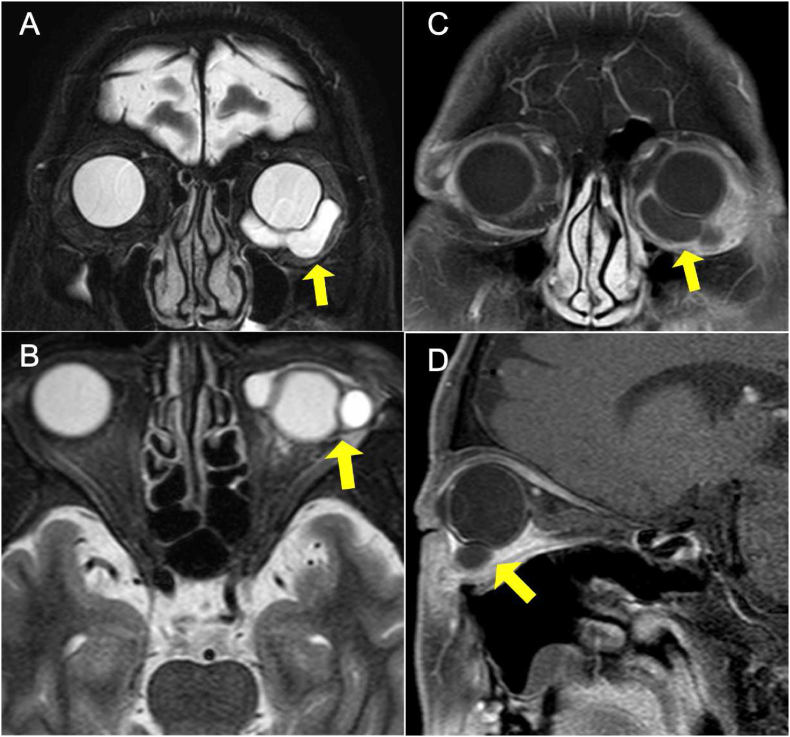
Contrast-enhanced T2-weighted MRI images of the orbit in (A; coronal) and (B; axial), and contrast-enhanced T1-weighted images (C; coronal) and (D; sagittal). There was no external ocular muscle hypertrophy or mass lesions in the bilateral orbits. Well-circumscribed buckle material found to have T1 hypointensity and T2 hyperintensity that was consistent with a fluid signal was observed around the left eye. The high intensity signal observed around the buckle material and subcutaneous in the left eyelid by T1-weighted fat-saturated post gadolinium suggested inflammation.
